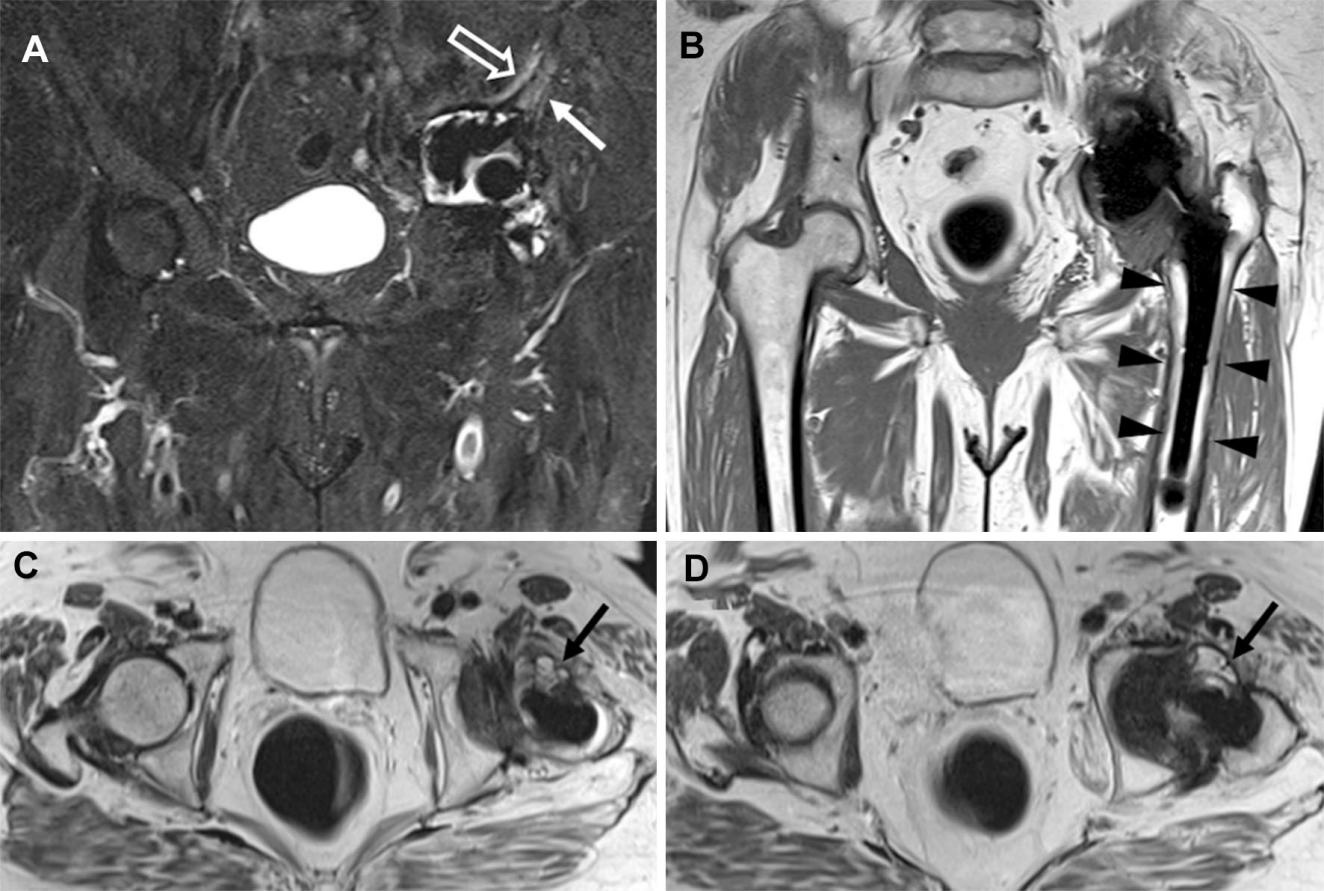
Pelvis MRI of a 77-year-old female with THA infection. Coronal STIR (A) shows periprosthetic acetabular bone edema (white arrow) and extracapsular edema (void arrow). No bone destruction is noted in the coronal T1-weighted image (B). Axial T2-weighted images (C and D) show effusion and synovitis
